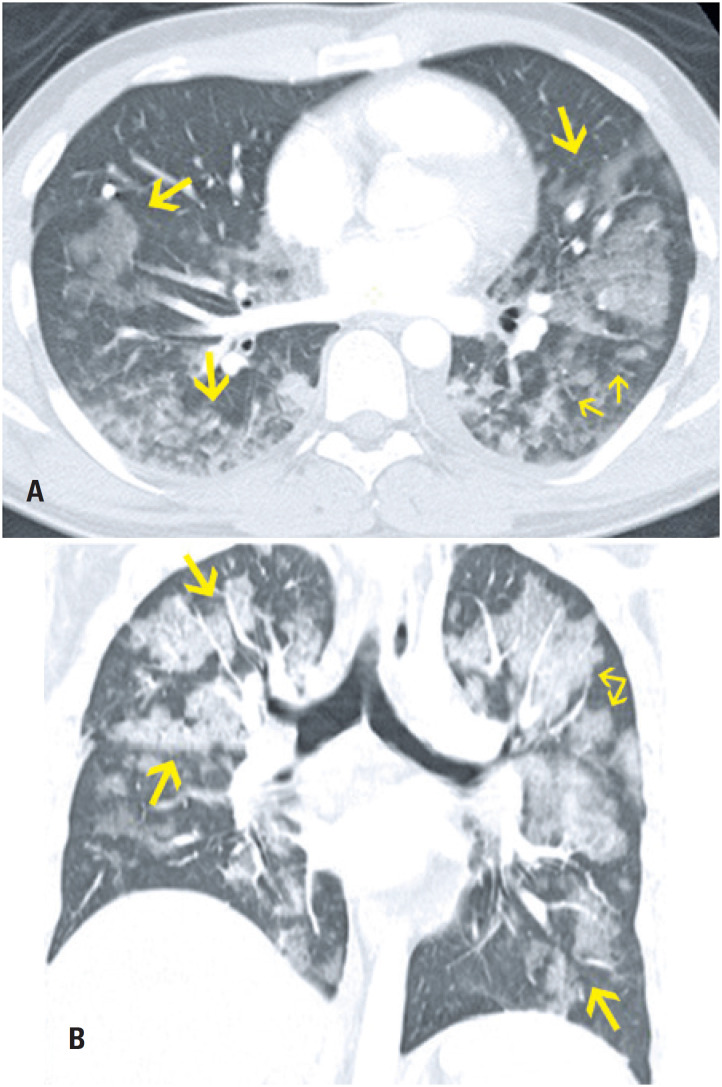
A 26-year-old male patient presented with sudden dyspnea after surgical correction of a tibial fracture using an external fixator. Computed tomography (A) Axial section and (B) Coronal reformation image: extensive ground-glass opacities and confluent alveolar consolidations predominating in the central regions of both lungs, compatible with fat embolism in the clinical context
